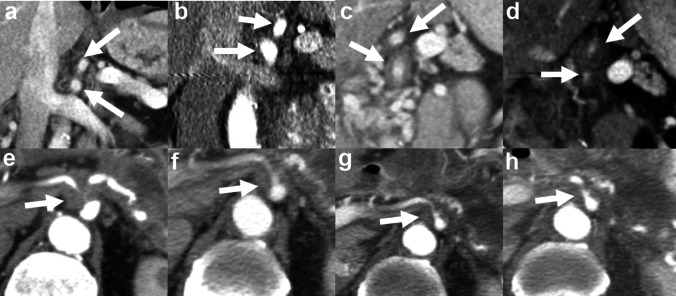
a–d Coronal contrast enhanced CT images of a patient treated with combination SBRT/chemotherapy over time with arrows noting changes in the celiac and superior mesenteric arteries, in the absence of disease progression. No vascular involvement of the celiac and superior mesenteric arteries pre-treatment (a) and 1 month post-treatment (b); encasement without stenosis of these vessels 6 months post-treatment (c); encasement with stenosis of these vessels 12 months post-treatment (d). e–h Axial contrast enhanced CT images of a patient treated with stand-alone chemotherapy over time with arrows noting the celiac artery. There is encasement of the celiac artery with stenosis pre-treatment (e) which does not change at 1 month (f), 6 month (g) and 12 month (h) post-treatment intervals
